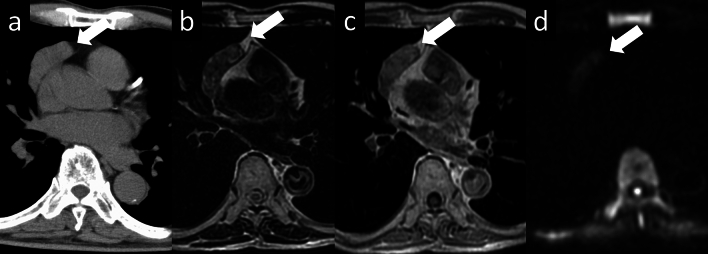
Thymoma (type B2). An 80-year-old man with type B2 thymoma. a Chest CT scan shows a 5-cm, slightly lobulated, prevascular mass (arrow). b–d MRI shows heterogeneous signal intensity on T2WI, heterogeneous enhancement on contrast-enhanced T1WI, and a low ADC value (1.13 × 10^–3^ mm^2^/s) (arrow) (b: T2WI, c: contrast-enhanced T1WI, d: DWI). CT, computed tomography; MRI, magnetic resonance imaging; T2WI, T2-weighted imaging; T1WI, T1-weighted imaging; ADC, apparent diffusion coefficient; DWI, diffusion-weighted imaging
